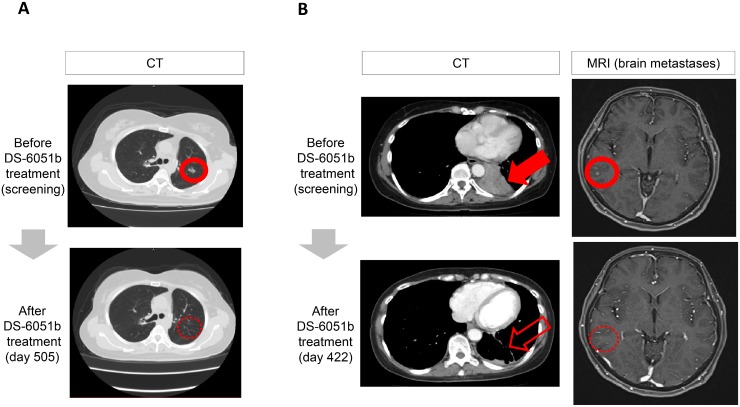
Computed tomography (CT) and magnetic resonance images (MRI) for objective responses (A) Patient #02: 400 mg QD, 43-year-old female, ROS1 + non-small cell lung cancer (NSCLC). Best response: partial response, crizotinib treatment-naïve. This patient showed a partial response from day 43 (showing a best response of −100% from day 127). (B) Patient #09: 400 mg QD, 61-year-old female, ROS1 + NSCLC. Best response: partial response, crizotinib treatment-naïve. This patient showed a partial response from day 44 (showing a best response of −79% from day 338) and her brain metastatic lesion disappeared. QD, once daily.
